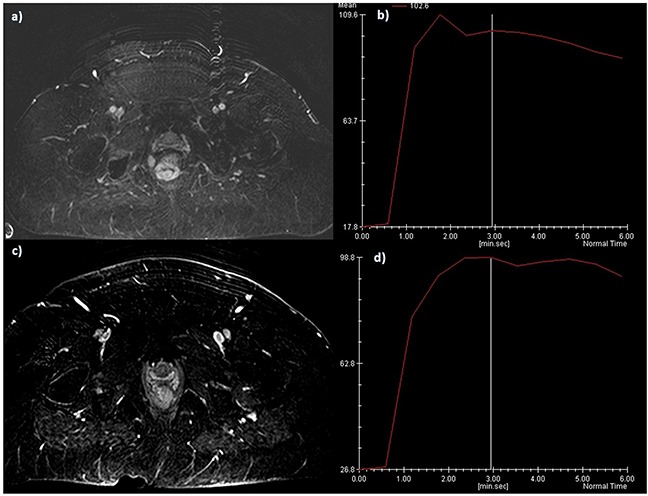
The same patient of Figure 3 and D, DCE-MRI and TIC Pre treatment evaluation: in a. subtraction image and in b. type 3 curve. Post treatment evaluation in c. subtraction image and in d. type 2 curve. Responder patient.
